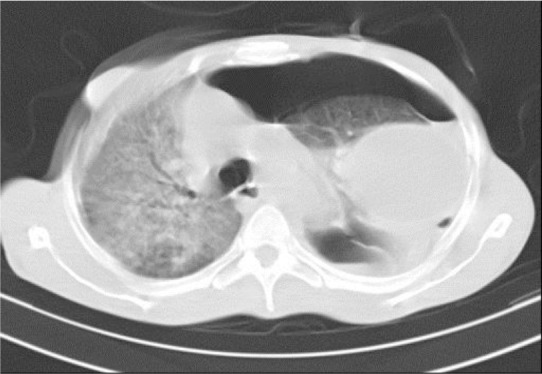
Tension pneumothorax in CT scan in association with severe pneumonitis.
